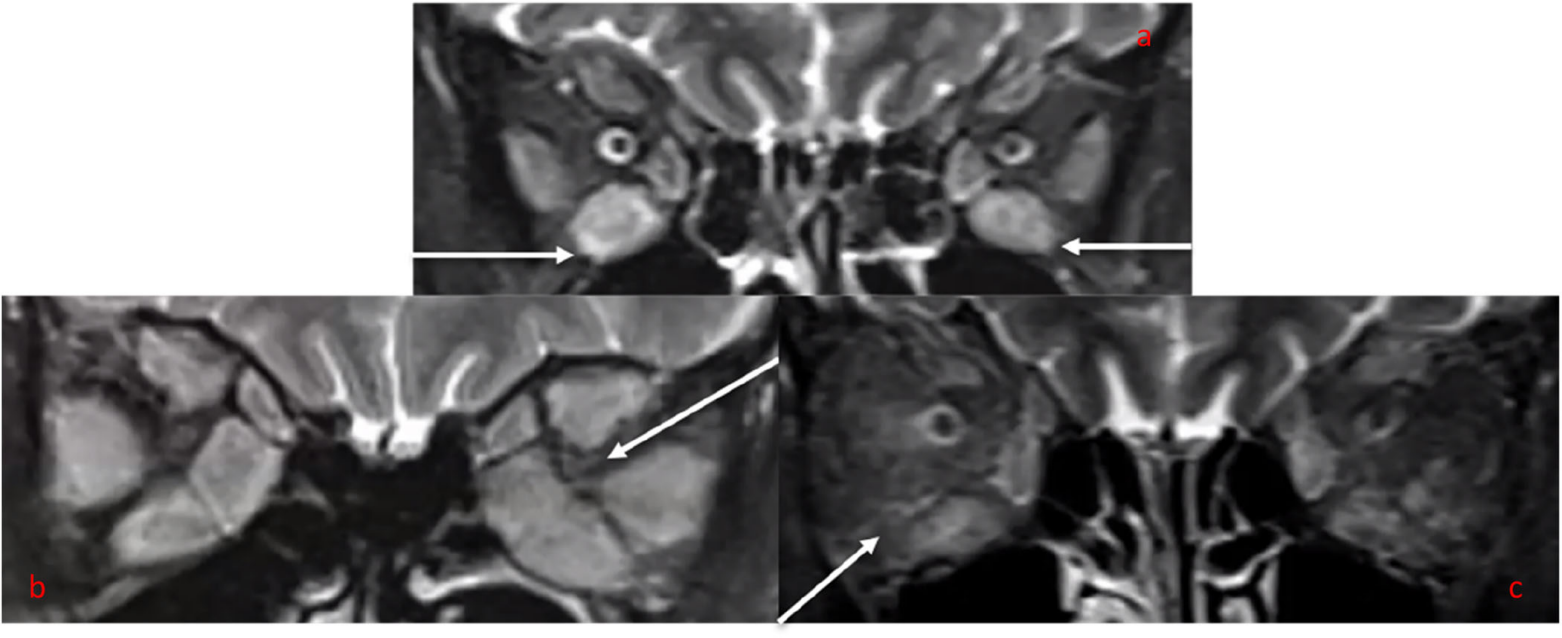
Coronal MRI STIR images (a) Muscle enlargement with elevated signal intensity in the inferior rectus muscles bilaterally in a patient with TED and no‐DON. (b) gross enlargement of all extraocular muscles bilaterally in a patient with TED and DON. This patient also has apical crowding (arrow) on the left side. (c): identifying right peri‐muscular fat signal intensity compared to the left orbit, which is less pronounced in a patient with TED.
